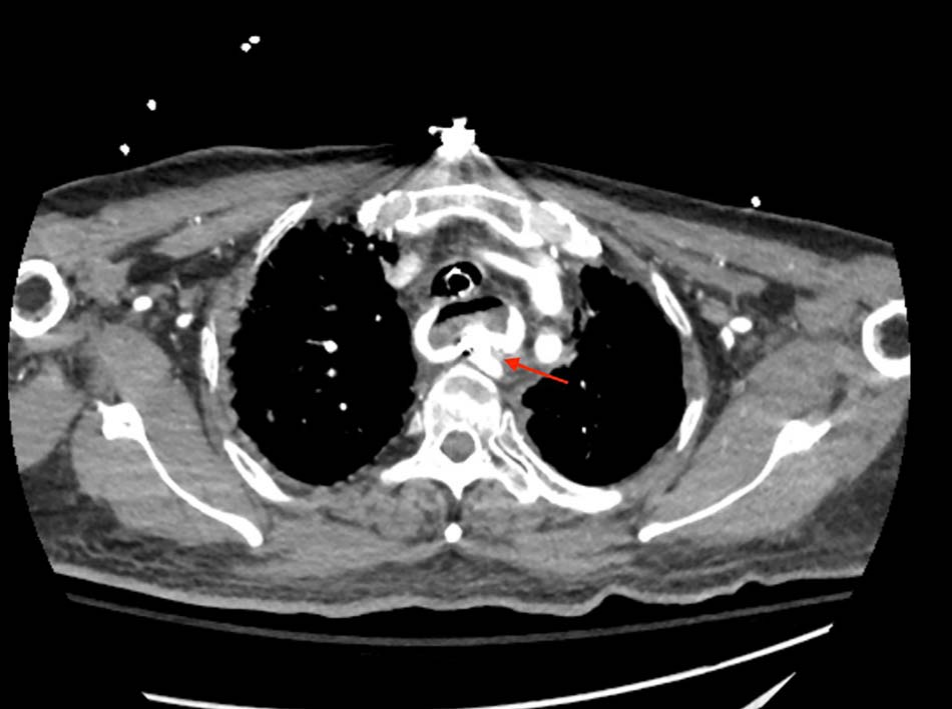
CT thoracic angiogram showing actively bleeding aberrant right subclavian artery perforating into the esophagus (red arrow).
